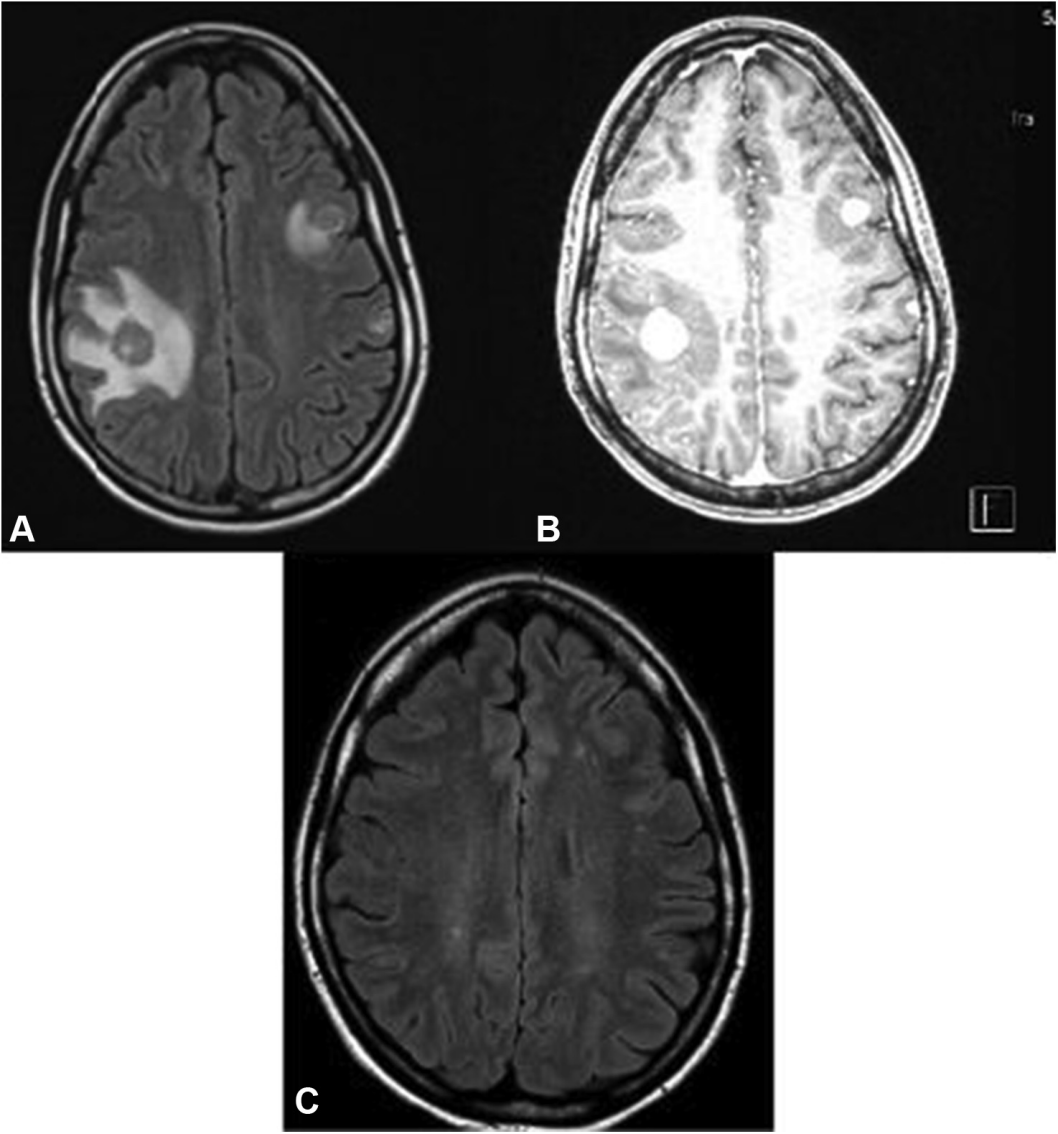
(*A*) MRI brain T2 flair at the time of diagnosis. (*B*) MRI brain with contrast at the time of diagnosis. (*C*) MRI brain with contrast 4.5 years after diagnosis. MRI, magnetic resonance imaging.
